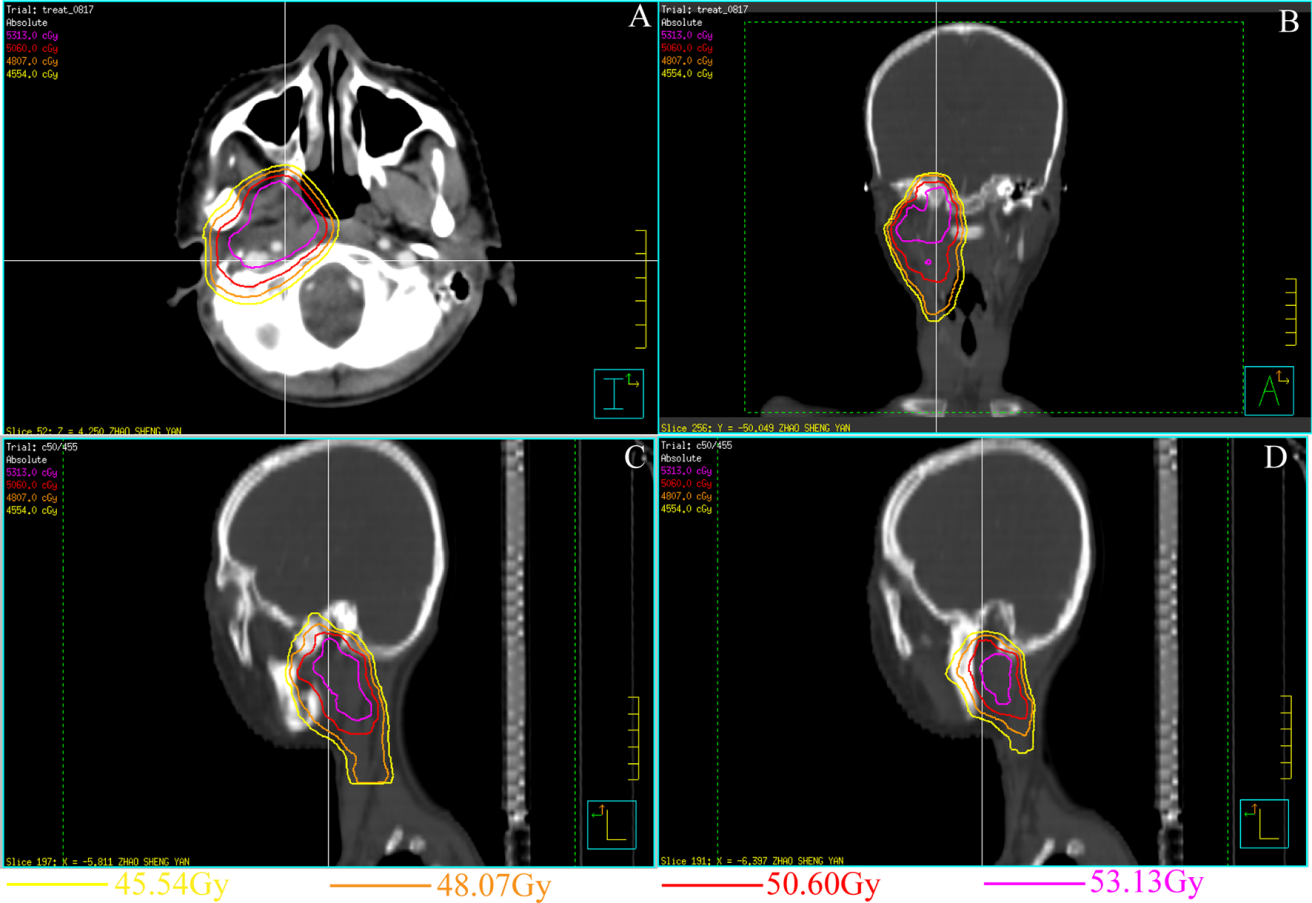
Treatment planning and set-up images for the rhabdomyosarcoma in the right pterygoid muscle 7 years ago (A–D). (A) Horizontal view CT image of the radiation area. (B) Coronal view CT image of the radiation area. The inner side of the right condylar area was exposed to high-dose radiation. (C, D) Sagittal view CT image of the radiation area.
